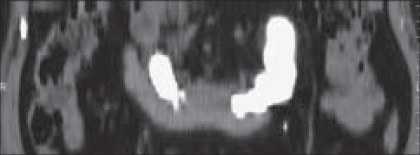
CT coronal confirming horseshoe kidney and bilateral stones
